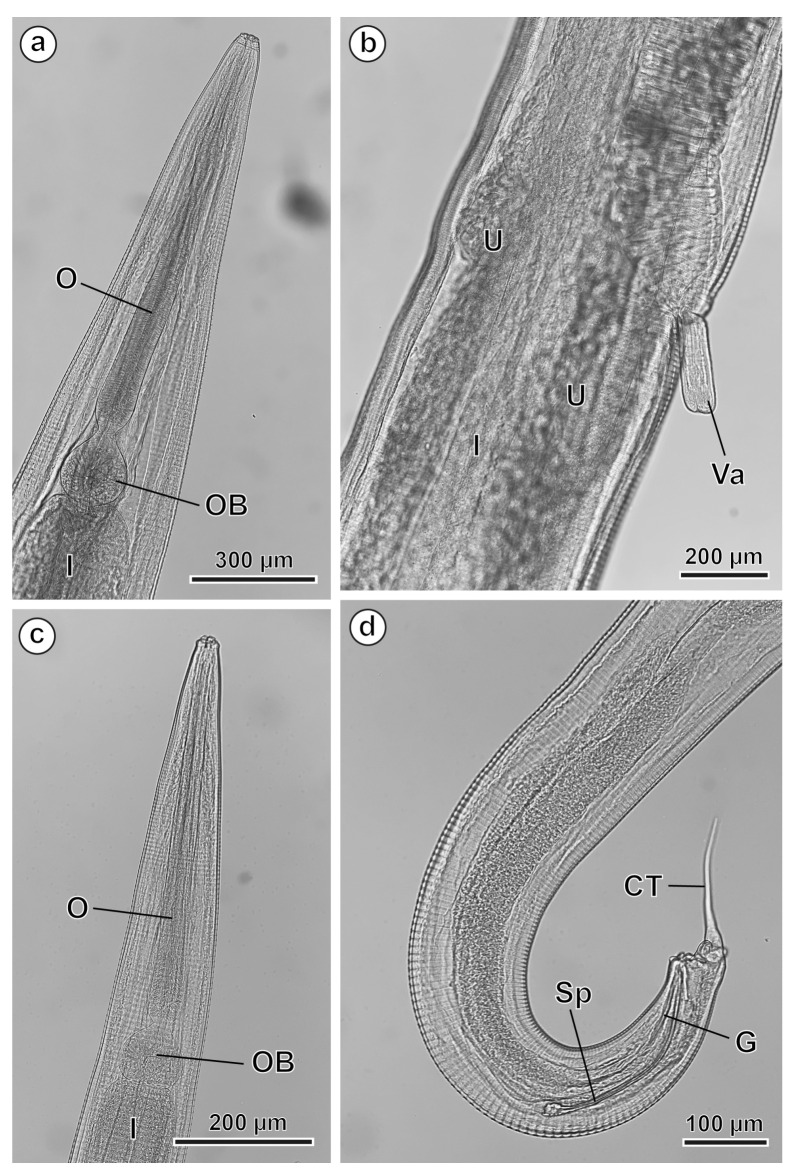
Hilgertia hilgerti*, LM. (a) Female anterior end showing the oesophagus and its oesophageal bulb. (b) Vulvar region showing the extroverted vagina. (c) Male anterior end showing the oesophagus and its oesophageal bulb. (d) Lateral view of the male posterior extremity showing the spicule, the gubernaculum and the caudal tip. (CT) caudal tip; (G) gubernaculum; (I) intestine; (O) oesophagus; (OB) oesophageal bulb; (Sp) spicule; (U) uterus; (Va) extroverted vagina; (1 to 4) pairs of caudal papillae.
